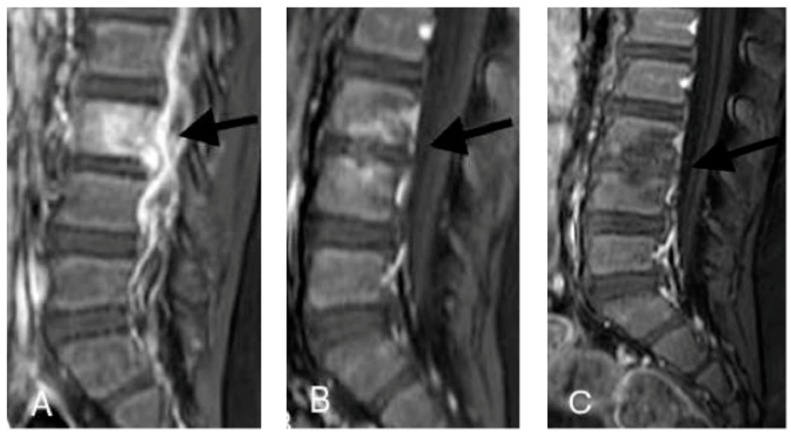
Lumbar spondylodiscitis, magnetic resonance (MRI) imaging of case 2. (A) Sagittal T1 post-enhancement image showing increased signal of the annulus L3–L4, of the opposite end-plates of L3 and L4, and of the adjacent soft tissues (black arrows); (B,C) at the same level as A, after therapy (1 month and 4 months later, respectively), there is a progressive decrease in the pathological enhancement (black arrows), which has almost disappeared in C. Residual irregularities of the end-plates of L3 and L4 are evident.
